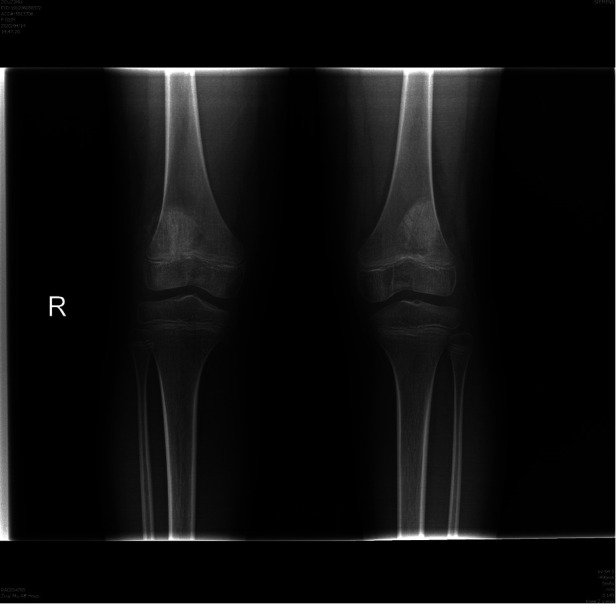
Post-operative AP radiograph of the both knees after removal of Kirschner and cerclage wires.
